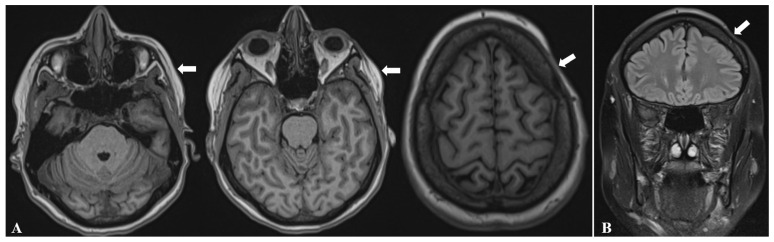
Skin and subcutaneous tissue atrophy involving the left hemiface and the ipsilateral scalp (white arrows), demonstrated on axial T1-weighted (A) and coronal T2-weighted brain MRI sequences (B).
